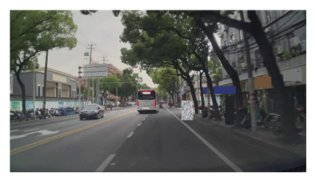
Highway	1200	Homogeneous and heterogeneous high-speed targets in a controlled-access setting	Trajectory tracking capability for distant targets with high Doppler shift
